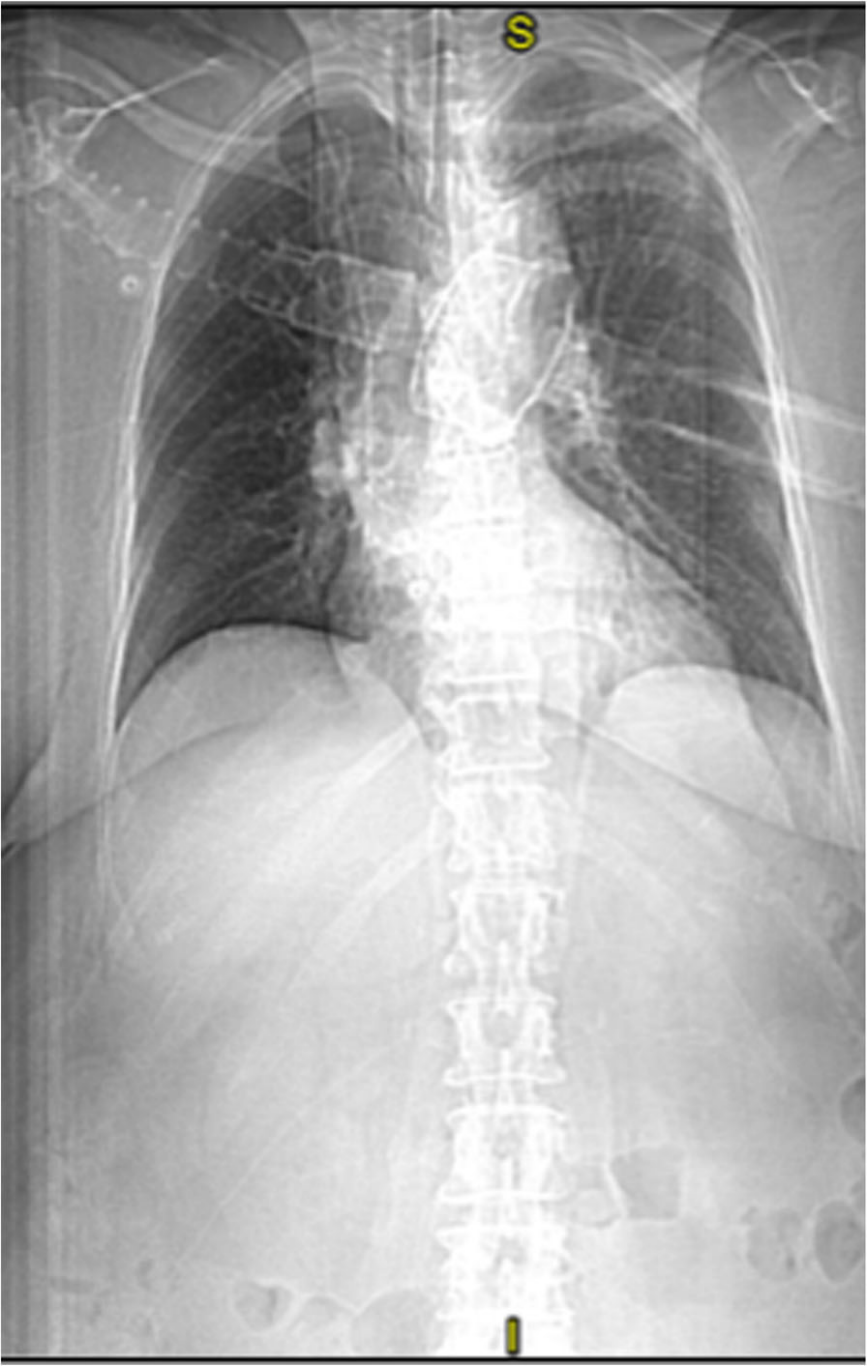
Lung computed tomography
